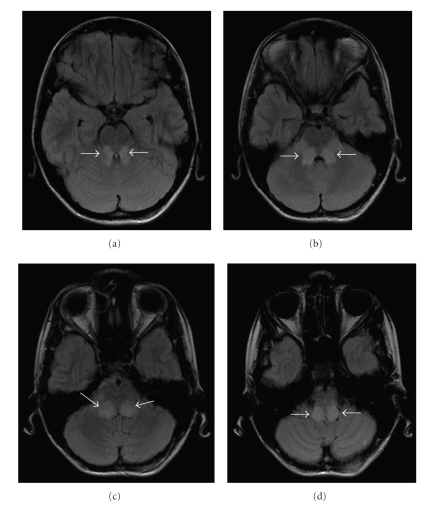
Axial fluid attenuation recovery (FLAIR) MR images show symmetric abnormal hyperintensity in the cerebellar tonsils and inferior, middle, and superior cerebellar peduncles.
